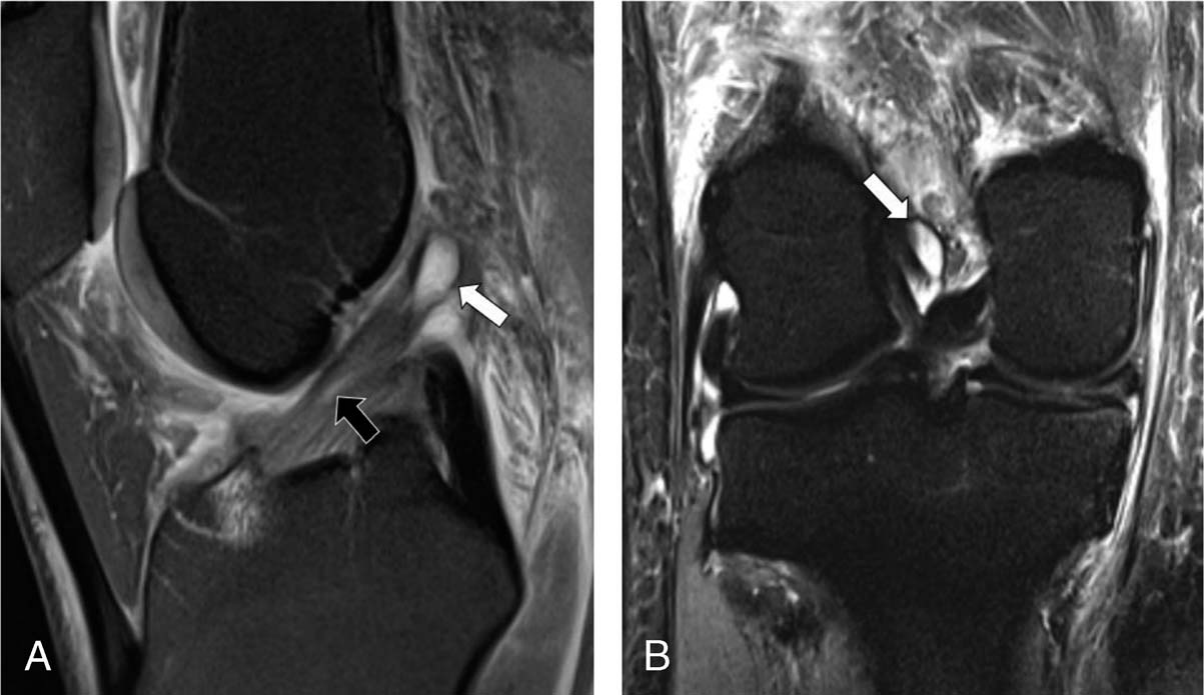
MRI of the right knee of a 43-year-old woman with knee injury 7 days earlier. Sagittal intermediate-weighted turbo spin echo image with fat suppression (A) and coronal short tau inversion recovery image (B) show diffuse and focal (black arrow) signal hyperintensity of the anterior cruciate ligament (ACL) indicative of mucoid degeneration and an intraligamentous ganglion cyst (white arrows) with otherwise continuous fibers in normal oblique orientation. Arthroscopic surgery confirmed mucoid degeneration of the ACL without fiber discontinuity. All 3 radiologists correctly diagnosed an intact ACL, whereas the DCNN erroneously classified the ACL as torn, representing a false-positive case.
